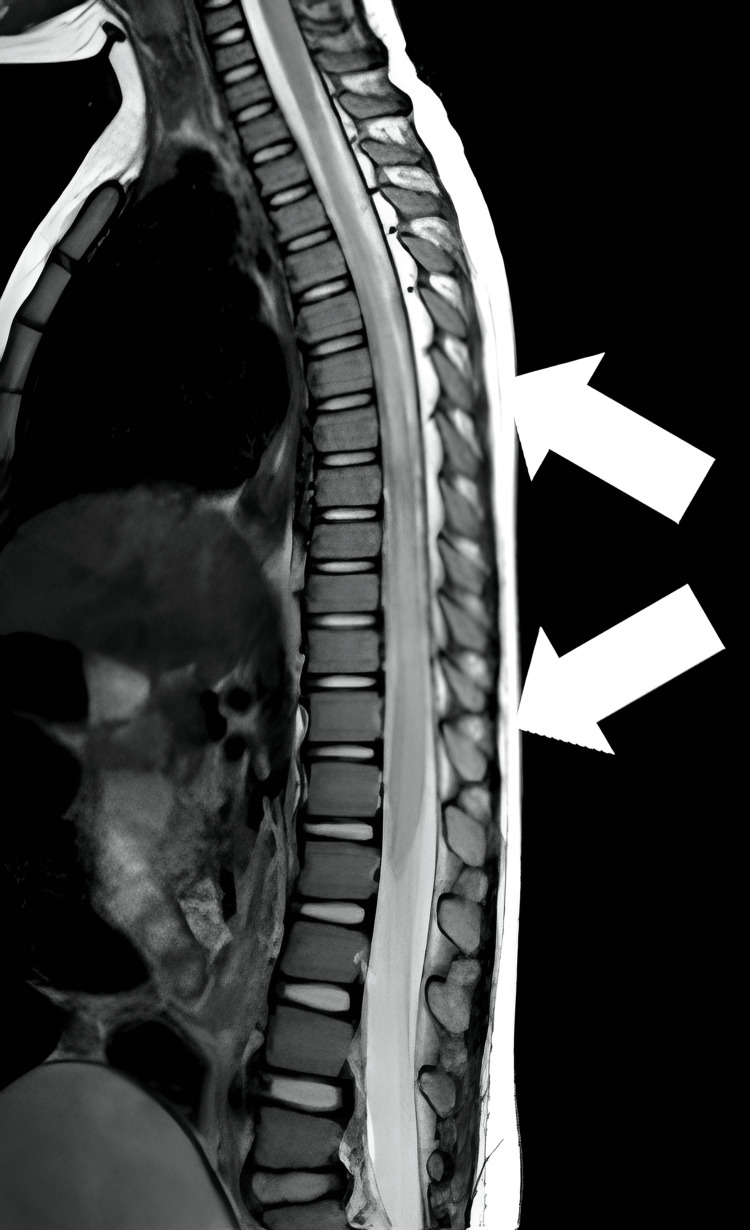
MRI of the whole spinal cord without IV contrast The image showed a long segment of abnormal T2W and FLAIR hyperintense signals in the thoracic cord from T5 to T11 MRI: magnetic resonance imaging; FLAIR: Fluid-attenuated Inversion-Recovery
